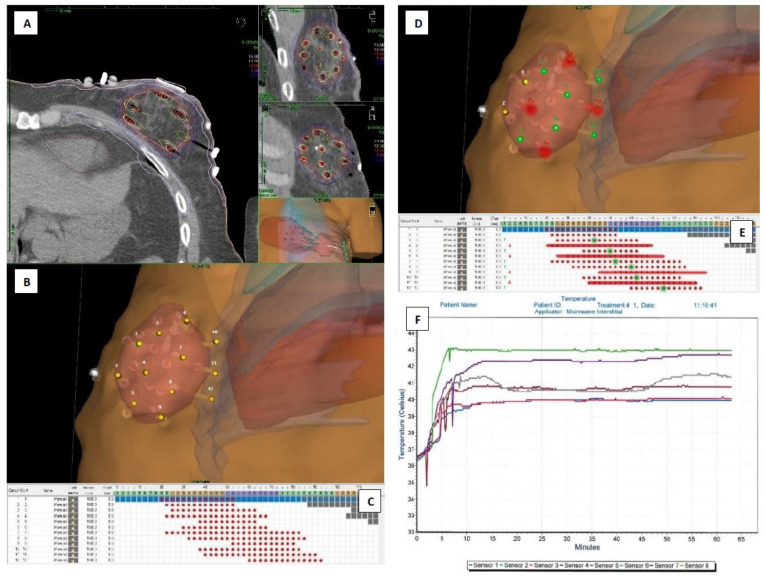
Case 1 planning and temperature measurement results—presentation: (A) cross-, sagittal- and frontal CT plane sections with optimized isodoses display, (B) applicator axis adjusted 3D view of the interstitial implant protruding the delineated tumor bed—salmon color, (C) source positions activated inside consecutive applicators—red dots, (D) applicators selected for 4 MW antennas (red) and 6 thermometers (green) insertion, (E) antennas active lengths adjusted to source positions—red lines, planned optimal positions for thermometers’ tips—green dots, (F) graphical presentation of the whole HT session temperature measurements with reference thermometer in green.
